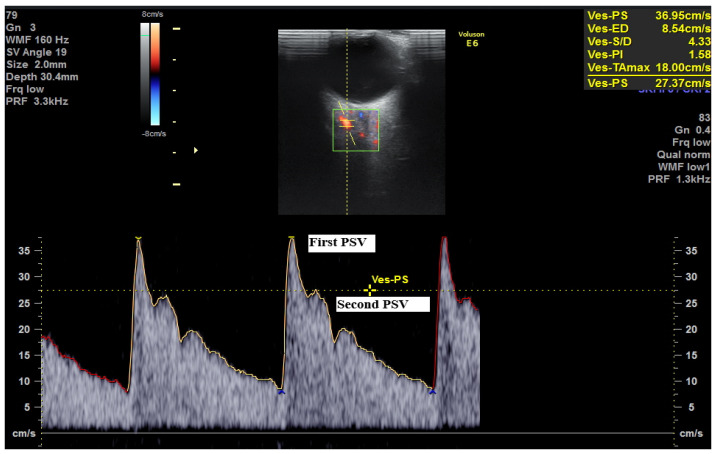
Ophthalmic artery Doppler.
